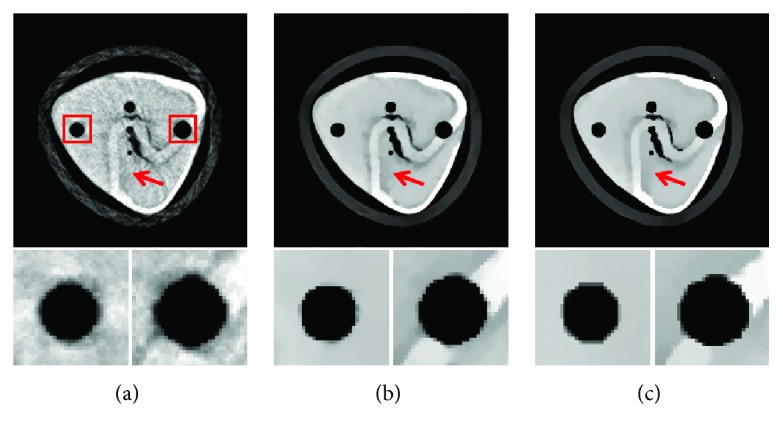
CT images of the modified buckwheat seed in experiment 3: (a) SART, (b) TV, and (c) TF-L0. The second row images are the close-ups of ROIs.
